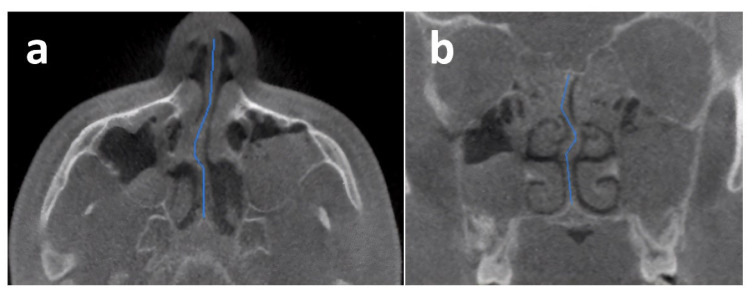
Axial (a) and coronal (b) CBCT scan showing the nasal septum deviation drawn with blue line.
